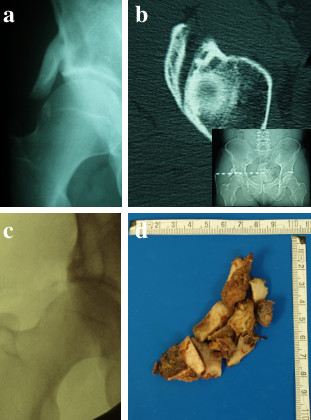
Conventional radiography (a) and computed tomography including scout view (b) shows a bony protuberance at the right anterior inferior iliac spine. Complete removal of the pelvic digit (c and d) restored the full range of motion within the patient's right hip joint together with complete relief of symptoms.
